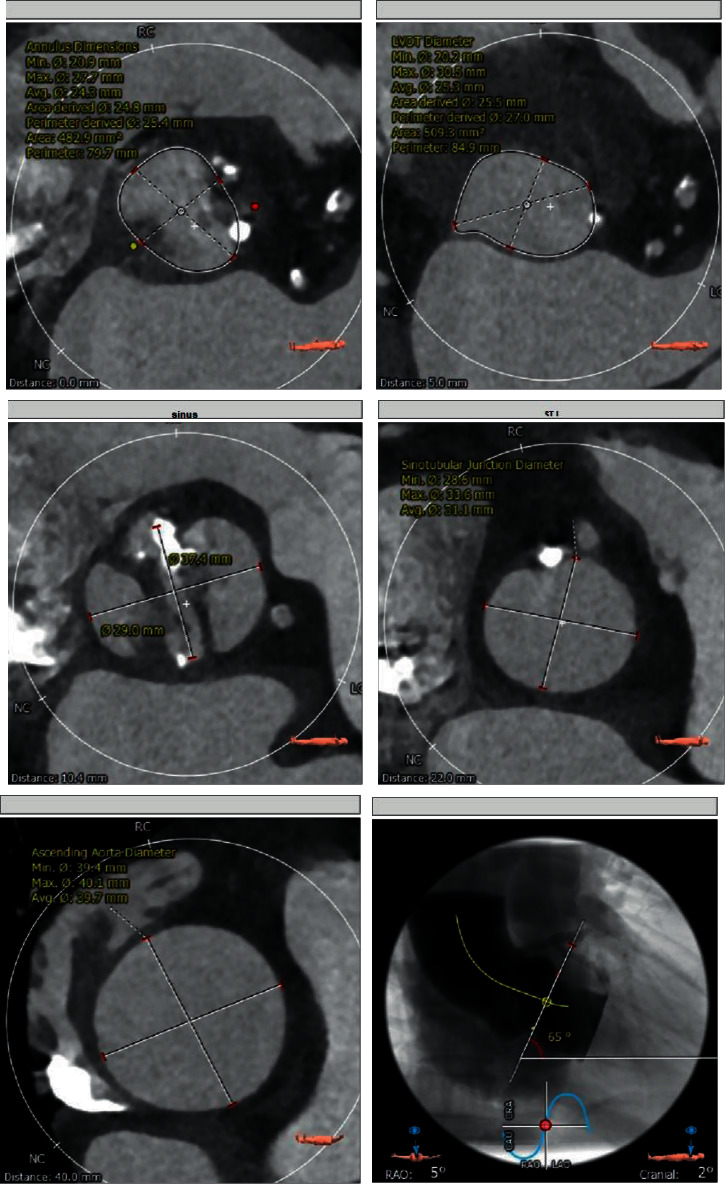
CT image and index measurement of patients.
